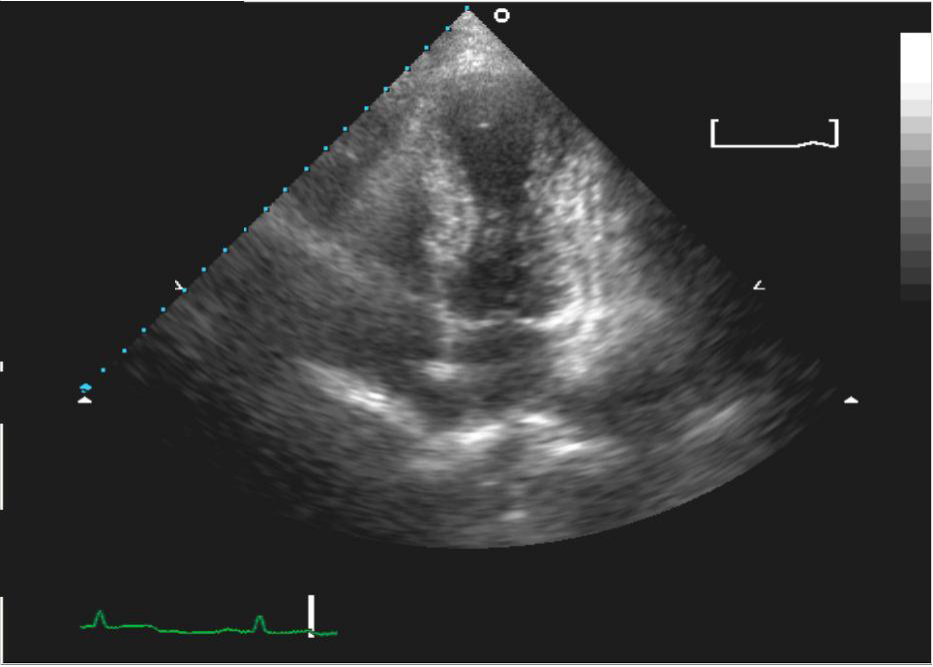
Echocardiography findings of apical hypokinesia in a four-chamber view.
